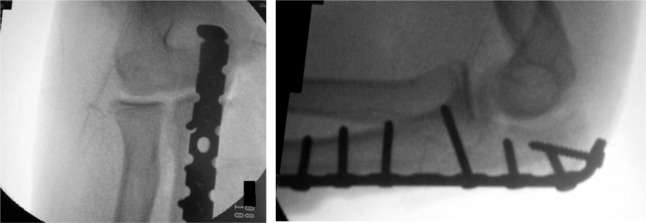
Radiograph post-plating proximal ulna under image intensifier showing good reduction.
